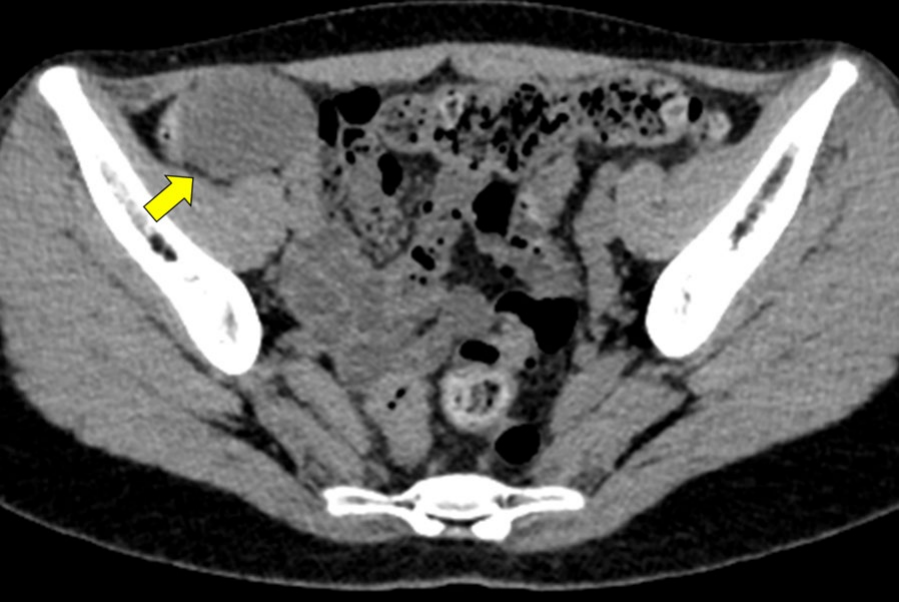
Abdominal contrast-enhanced CT. CT shows a 56 × 35-mm cystic mass with no enhancement in the ileocecal area (arrow)
